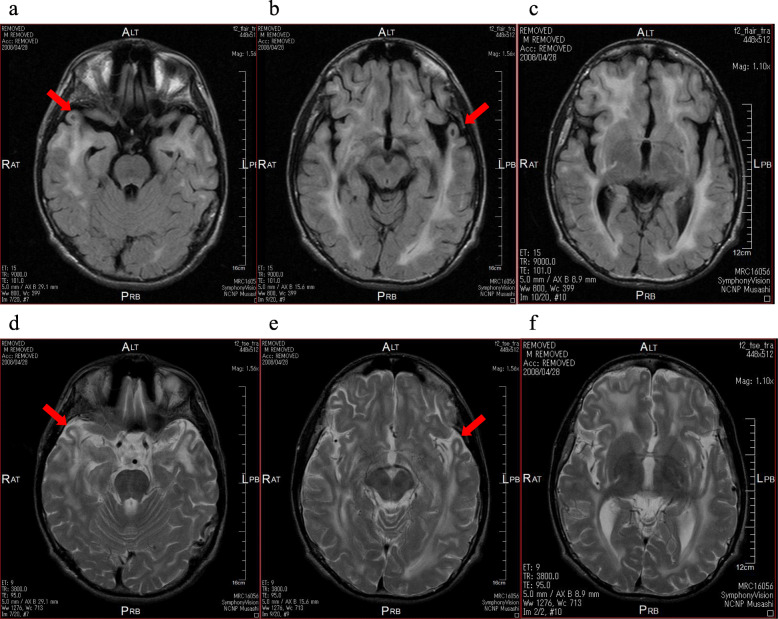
Brain MRI on admission at 17 years of age. a, b, and c: axial FLAIR images. d, e and f: axial T2-weighted images. Diffuse high signal intensity is seen in bilateral cerebral white matter. Frontal and temporal subcortical areas are predominantly involved. Arrows indicate characteristic subcortical cysts in both temporal lobes
